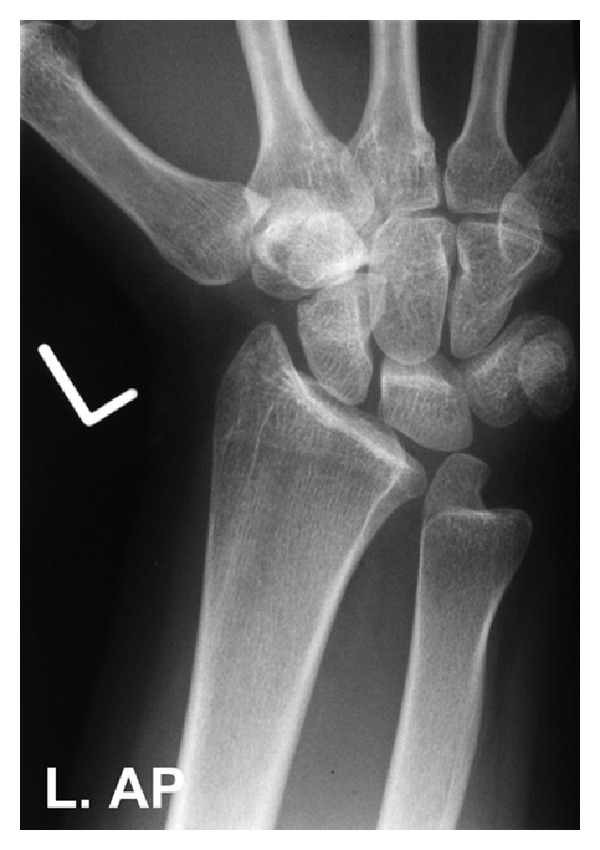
AP radiograph of the contralateral left wrist demonstrating the abnormal appearance of the ulnar styloid in supination. The lie of the ulnar styloid in supination is identical to that of the injured right wrist.
